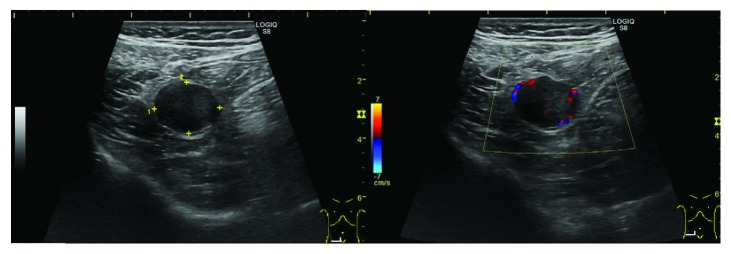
Abdominal ultrasonography showed that a well-demarcated hypoechoic mass of 22 mm × 18 mm × 18 mm. There were cystic area and blood flow sign.
